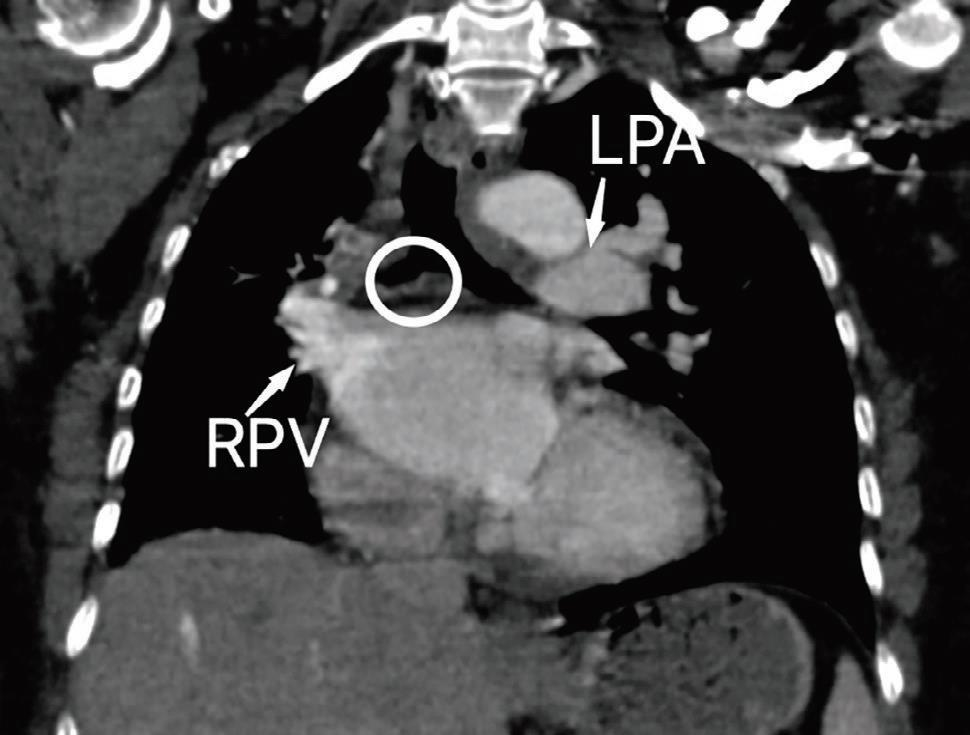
Coronal CT reconstruction image of CT chest in venous phase demonstrating the absence of right main pulmonary artery (white circle) and prominent right pulmonary vein (RPV). LPA—left pulmonary artery.
